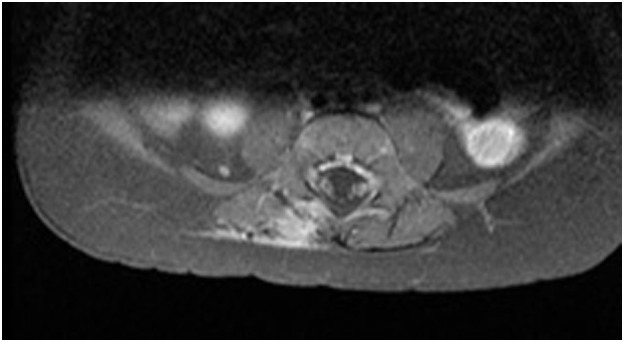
MRI after surgical excision (axial).
